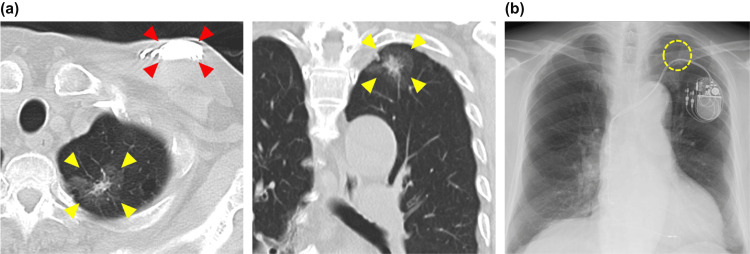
Pretreatment imaging studies (a) Computed tomography (CT) scan. The yellow arrow indicates the left upper lobe lung cancer. The lesion comprises a central solid component with a maximum diameter of 1.2 cm, surrounded by a ground-glass opacity. The red arrow indicates the pacemaker. (b) Chest X-ray. The yellow dashed line shows the left upper lobe lung cancer lesion (not clearly identifiable on radiography but determined based on CT imaging). The tumors and pacemakers were relatively close to each other.
